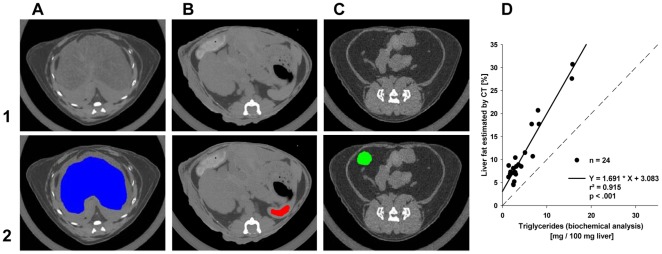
Quantification of hepatic fat by CT. Selected areas of liver (A; blue), spleen (B; red) and WAT (C; green) for determination of mean HU values. upper panel (1): raw gray scale scan slices, lower panel (2): selected organ parts used in calculation of liver fat. (D) Relationship between amounts of intrahepatic fat isolated and quantified with biochemical analysis and estimations by computed tomography. Dashed line – identity line, r^2^ - coefficient of determination.
